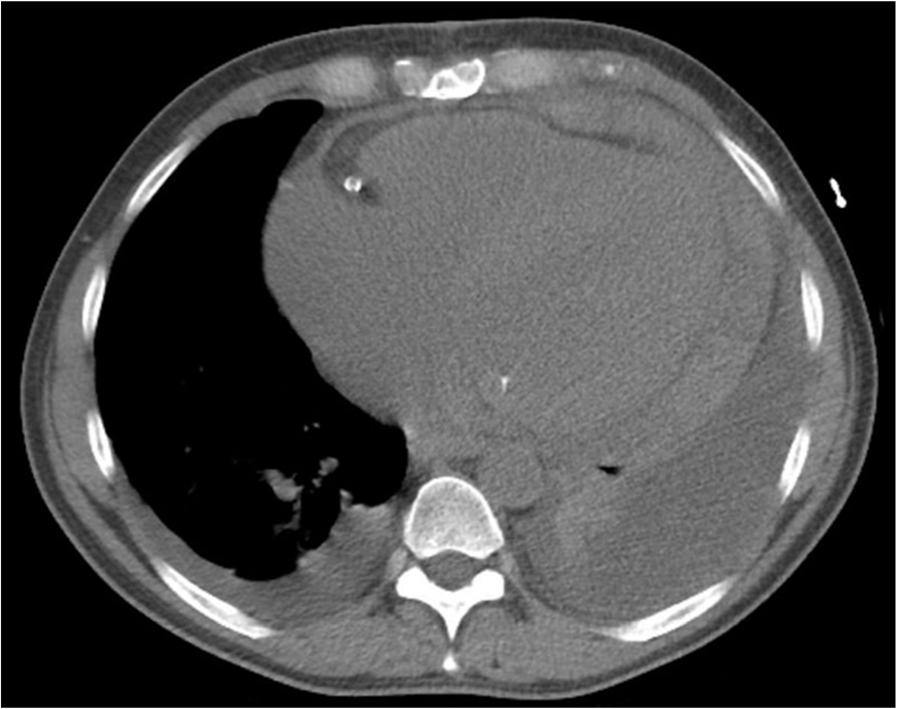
Non-contrast axial image from a dedicated aortic CT through the lower chest, showing hemopericardium in a 43-year-old male who being evaluated for congestive heart failure and low ejection fraction, following aortic valve surgery
